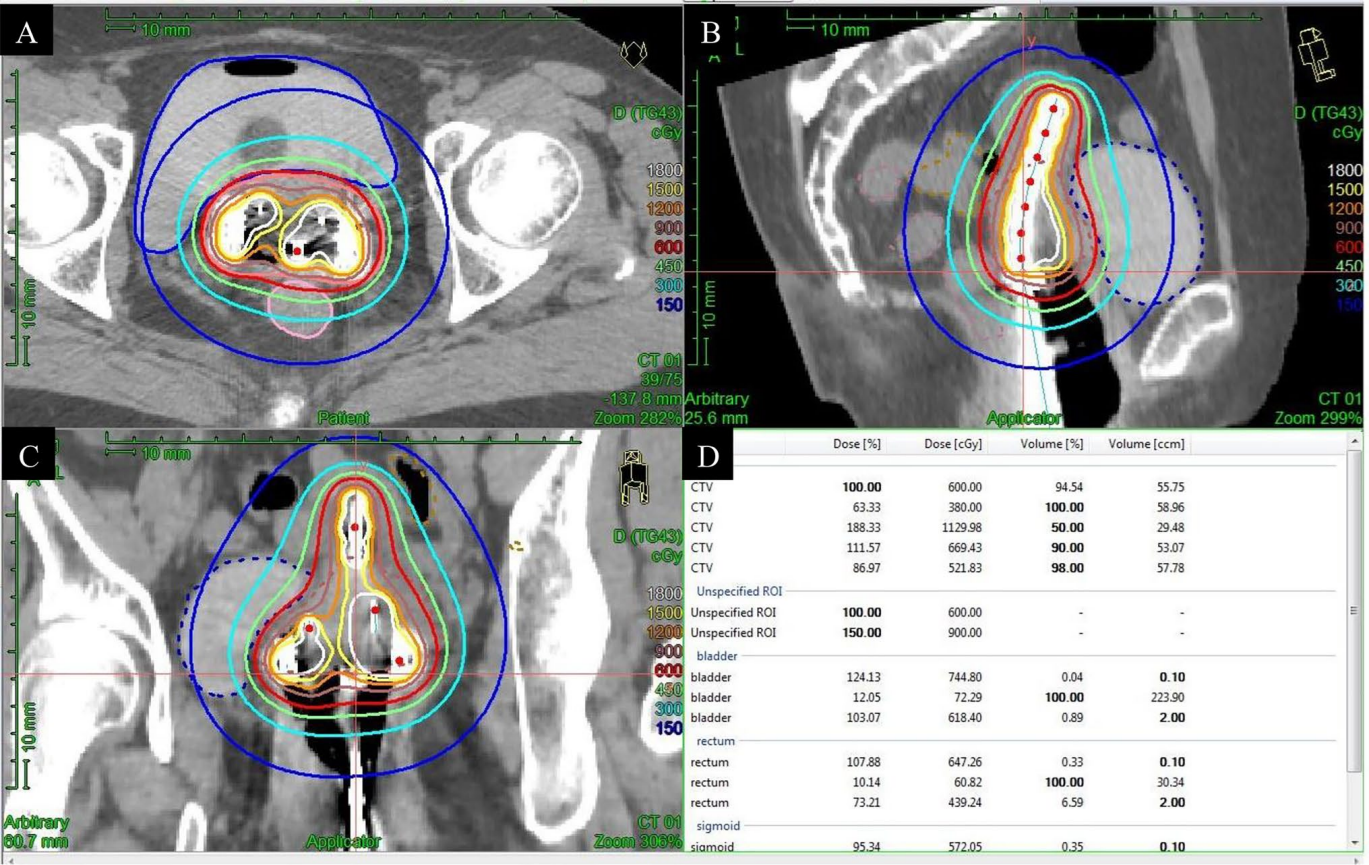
CT image-guided brachytherapy treatment plan visualization and dosimetry of squamous cell carcinoma in a woman in her sixties. The figure shows the dose distribution (cGy) surrounding the applicator in the A axial, B sagittal, and C coronal views. Table (D) summarizes the dose and volume parameters for the clinical target volume (CTV) and organs at risk. Key parameters included the dose [%] and dose [cGy] delivered to the specified percentage of the region of interest (ROI), as well as the volume [% and ccm] covered. Specific data is included for various CTV sub-regions (DTG43 and CASY) and organs at risk, including the bladder, rectum, and sigmoid colon
